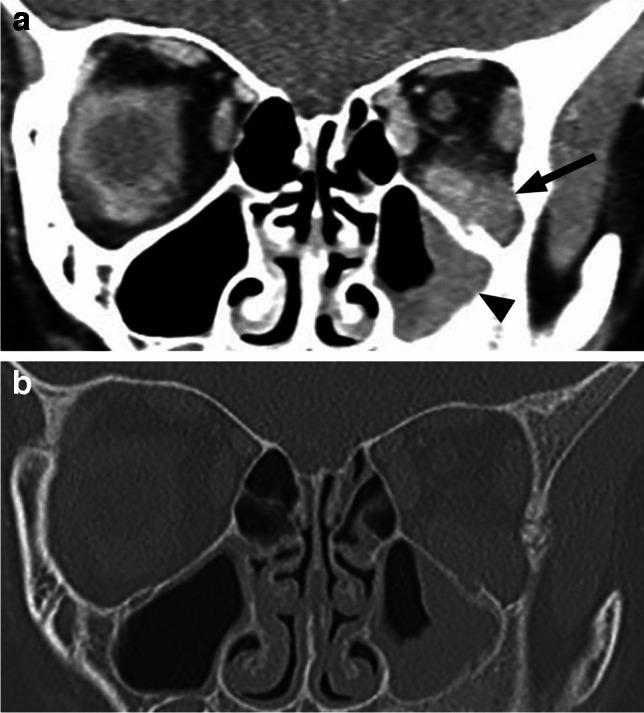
Orbital myeloid sarcoma. Coronal postcontrast CT in soft tissue (a) and bone (b) window and algorithm shows an infiltrative soft tissue mass (arrow) in the inferior left orbit extending to the left maxillary sinus (arrowhead) without bone erosion in this patient with AML
